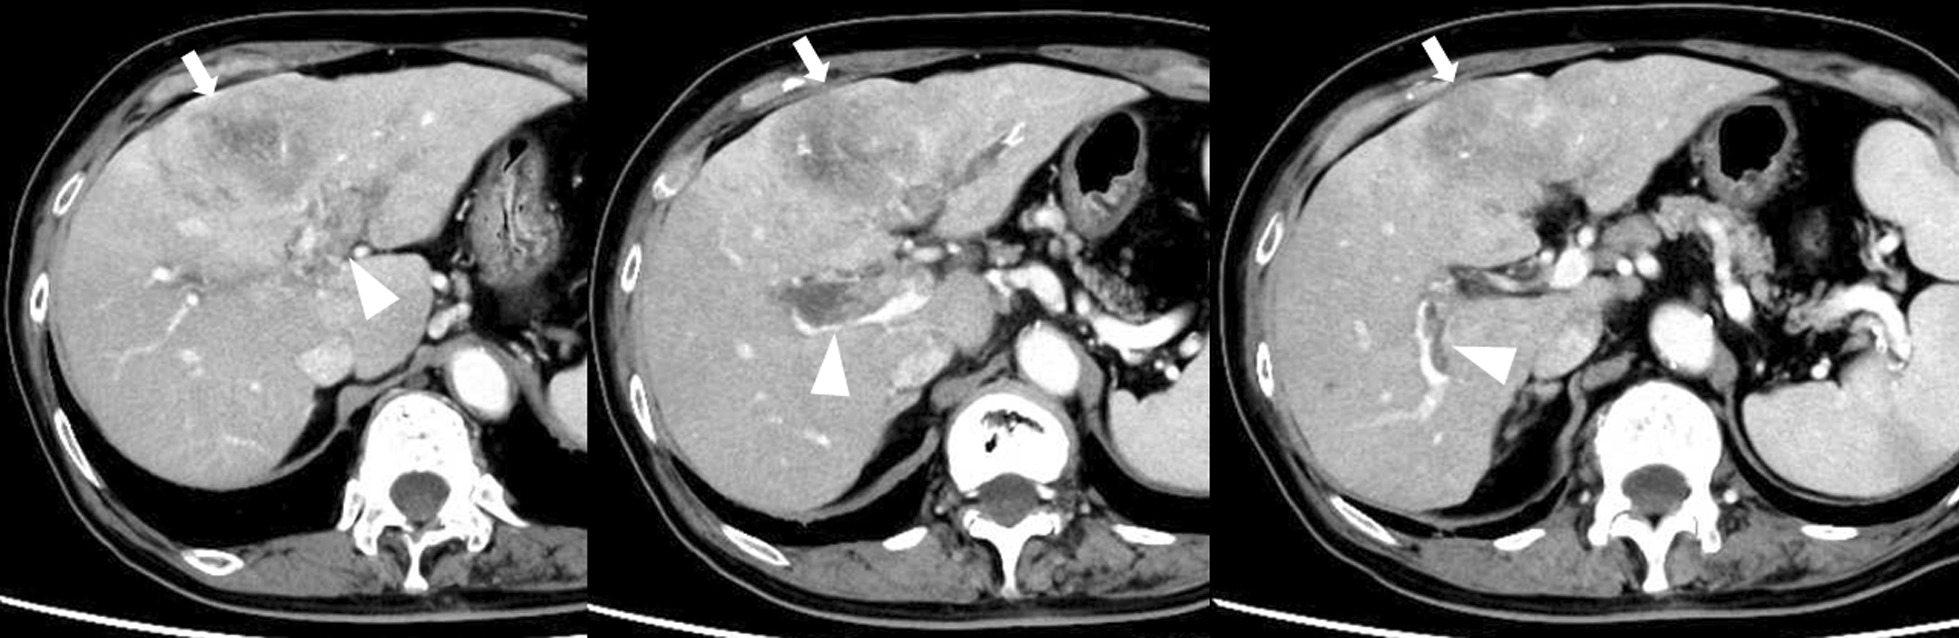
Abdominal computed tomography revealing a huge hepatocellular carcinoma located at segment 4 (white arrow). The portal vein tumor thrombus has spread from the adjacent portal vein to the left portal trunk, main portal trunk, through to the contralateral right portal trunk (arrowhead). The tip of the portal vein tumor thrombus has invaded into the bifurcation of the anterior and posterior branches; the anterior branch is filled with tumor thrombus, while the posterior branch is filled with tumors or blood thrombi
